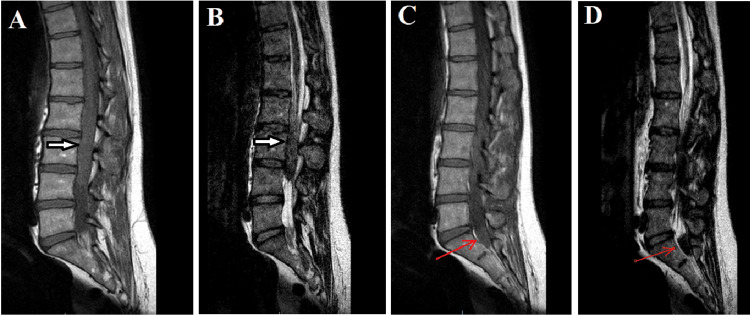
Preoperative MRI demonstrating lesions A: Sagittal T1-weighted MRI of the first lesion (white arrow). B: Sagittal T2-weighted MRI of the first lesion (white arrow). C: Sagittal T1-weighted MRI of the second lesion (red arrow). D: Sagittal T2-weighted MRI of the second lesion (red arrow). MRI: magnetic resonance imaging
